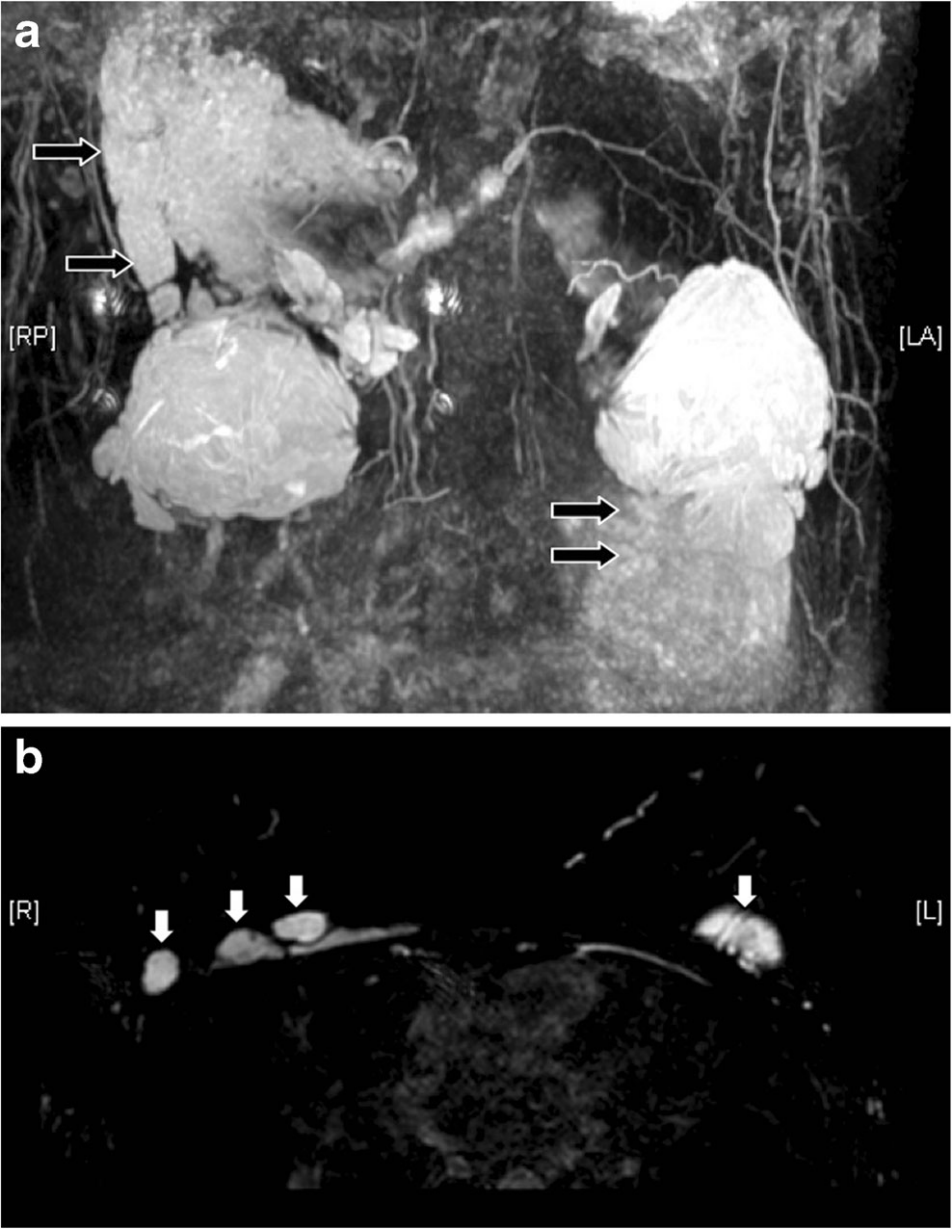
Free silicone at chest wall. Reconstructed T2 coronal MR image with MIP (a) reveals extensive silicone leakage (black arrows) from bilateral silicone gel-filled implants. The free silicone has identical signal with the silicone gel inside the implants. On T2-weighted, fat-saturated axial image (b) discrete free silicone foci are seen over the chest wall (white arrows)
